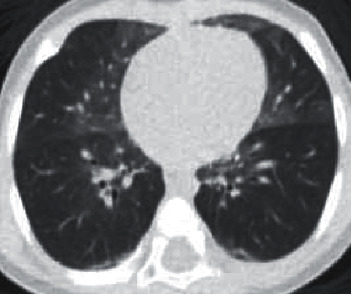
CT chest axial view shows groundglass opacities primarily involving right middle lobe and lingula.
